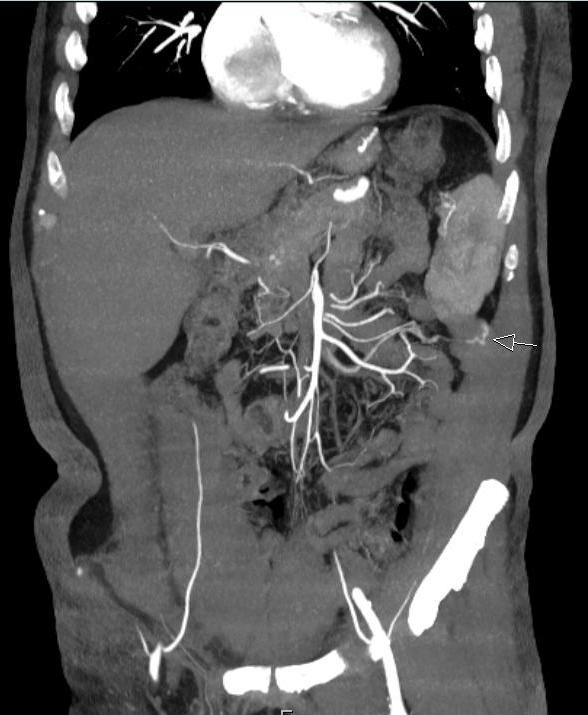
CTA - Coronal reconstruction with a slab of 1 cm. Abnormal vessel (AVM) (arrow) from a small jejunal branch of SMA.
